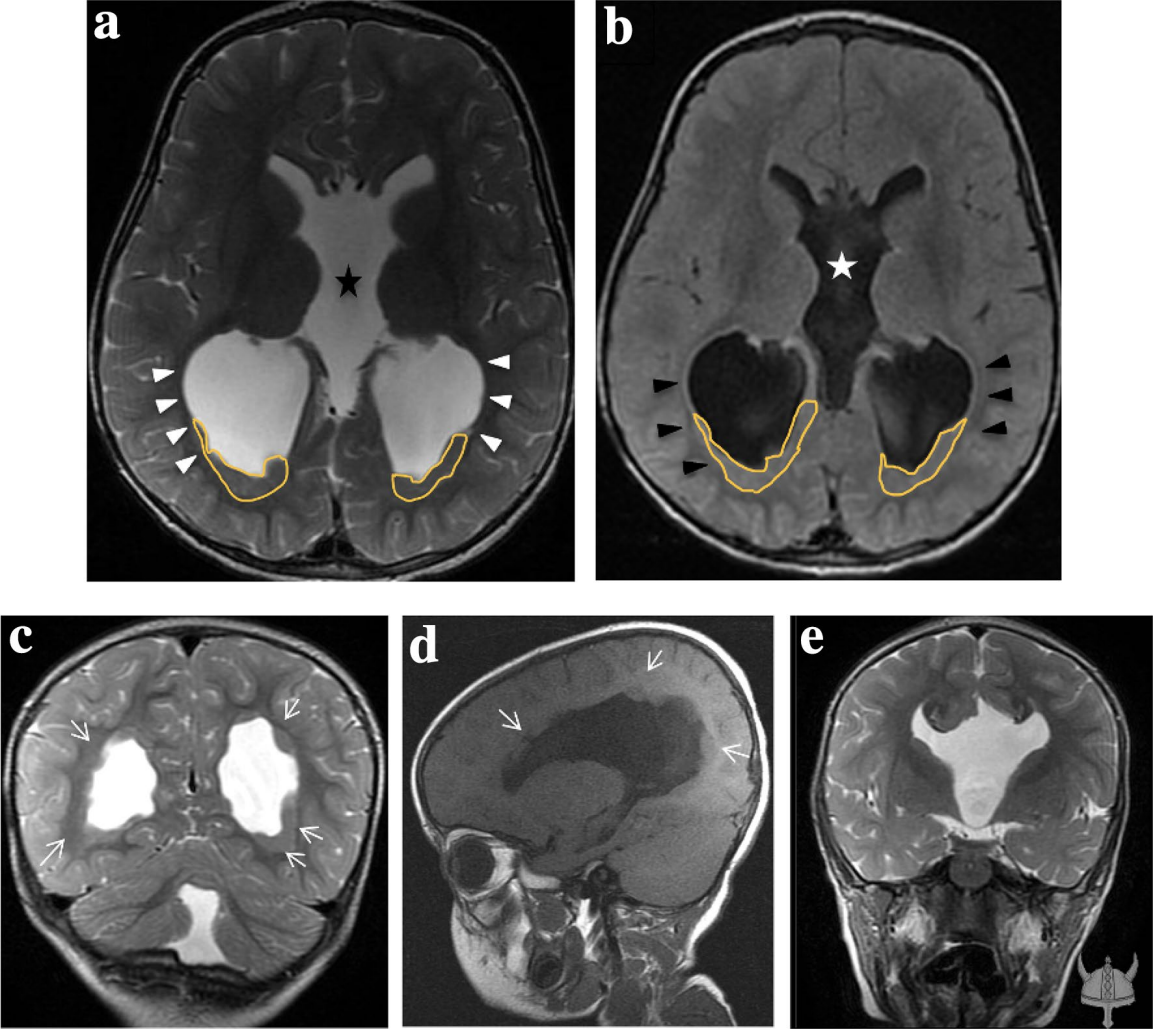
(ID 108): Agenesis of the corpus callosum and cavum septum pellicidum, ventriculomegaly and cerebellar vermis atrophy in patient with bilateral periventricular nodular heterotopia (PVNH)*. a, b Non-contrast enhancement axial T2-weighted MR image a and T1-weighted MR image b shows enlargement of the ventricles in particular the images show dilatation of the third ventricle (star) and lateral ventricles with widely spaced parallel bodies (arrowheads) in patient with bilateral periventricular nodular heterotopia (white lines). c Unenhanced T2-weighted MR coronal image show bilateral periventricular nodular heterotopia (PVNH) (arrows), enlarged lateral ventricles and cerebellar vermis atrophy. d Unenhanced T1-weighted MR midsagittal image show dysmorphic ventricular system and periventricular nodular heterotopia (arrows). e T2 -weighted MR coronal image shows the “Viking helmet appearance” refers to the morphology of the lateral ventricles in the coronal plane in patients with dysgenesis of the corpus callosum. The cingulate gyrus is everted into narrowed and elongated frontal horns
